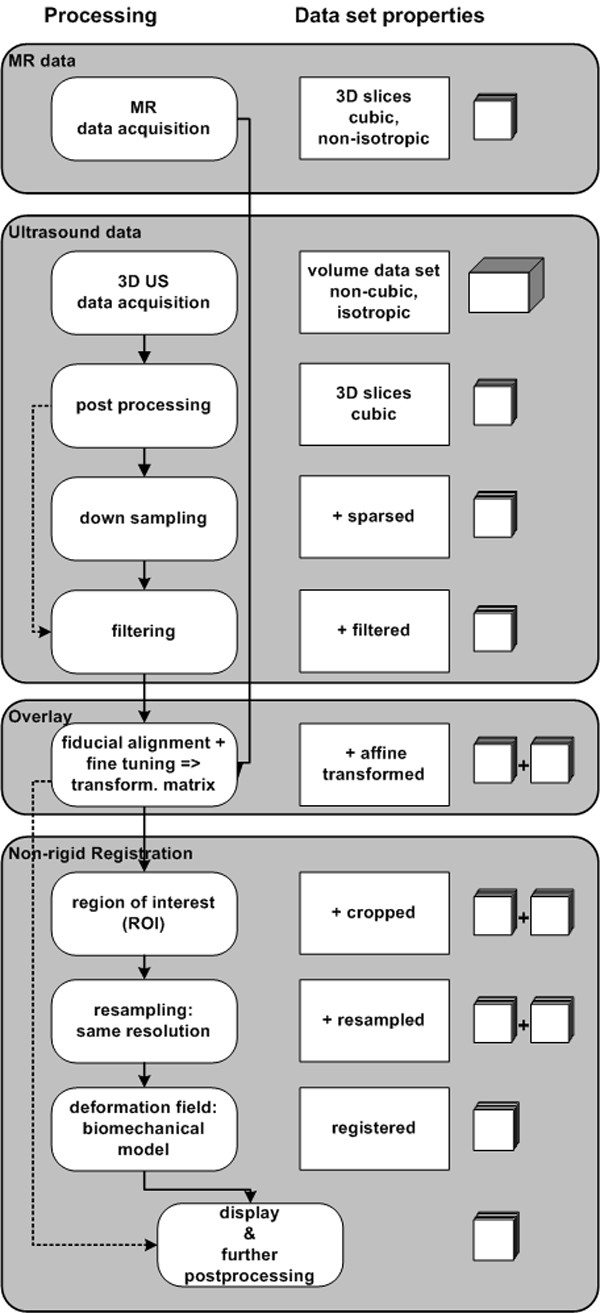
Strategy to register. Strategy to register MR and 3D ultrasound image data sets. Solid lines display the strategy shown in this paper. Dashed lines symbolize optional ways which were followed but which show no further relevant and new details. The symbols on the right refer to the format of the data sets: The series of squares stands for a series of parallel slices and the cube is for a volume block format.
